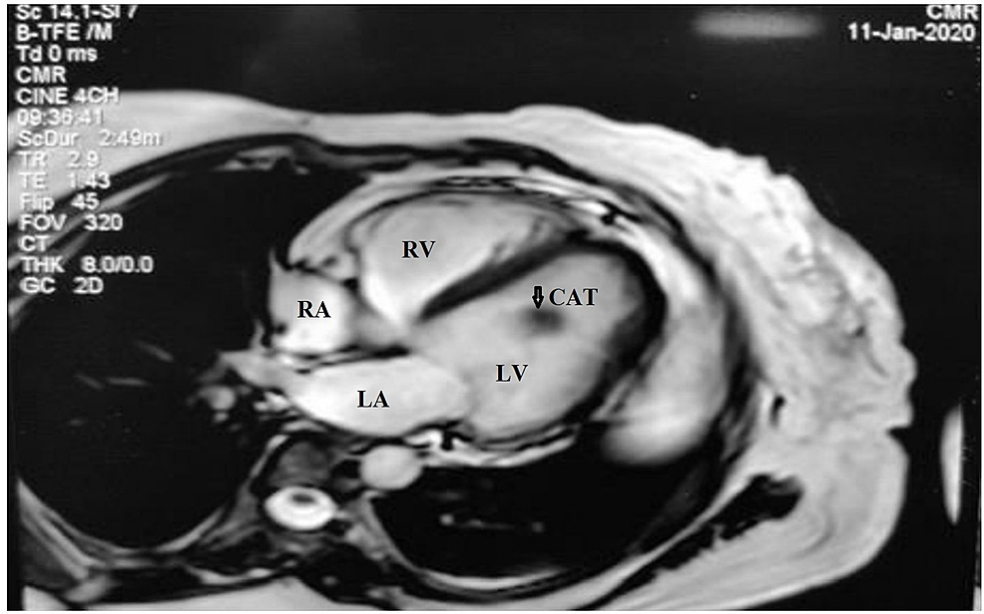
Cardiac T2-weighted magnetic resonance imaging showing isointense mass in the LV attached to endocardium of the interventricular septum through a narrow pedicle. LV, left ventricle; LA, left atrium; RA, right atrium; RV, right ventricle; CAT, calcified amorphous tumor.
